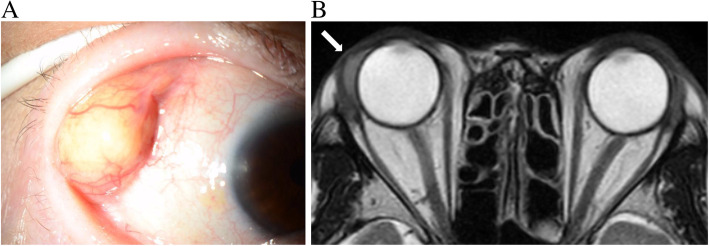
a. An image of anterior segments. Well-demarcated mass mimicking herniated orbital fat was observed in the superior-temporal quadrant. b. Magnetic resonance imaging of the orbit. A hypo-intense lesion on T2-weighted images at the temporal peribulbar area was found
